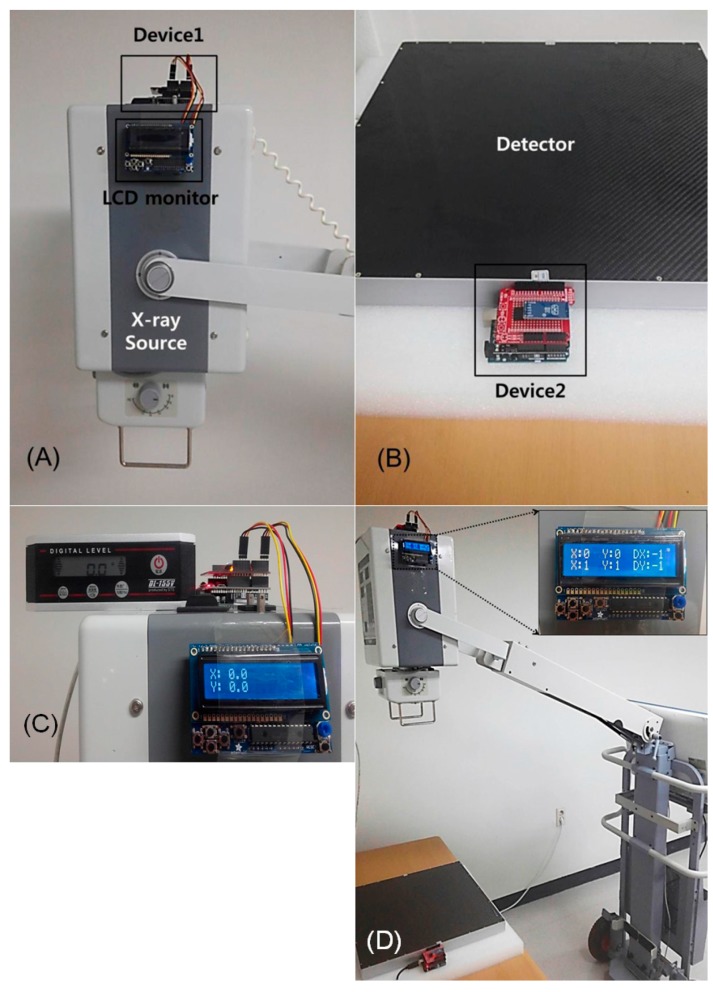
Details of the device for obtaining the SDA, which was attached on an X-ray source (A) and a detector (B). Photography showed the initial setting for obtaining the angle degree using the SDA device and digital angle meter (C). The ‘0.0°’ value on the SDA device was accordant with a ‘0.0°’ reference value on the digital angle meter. The measured angle degree using PDR with SDA device displayed on a LCD monitor (D).
